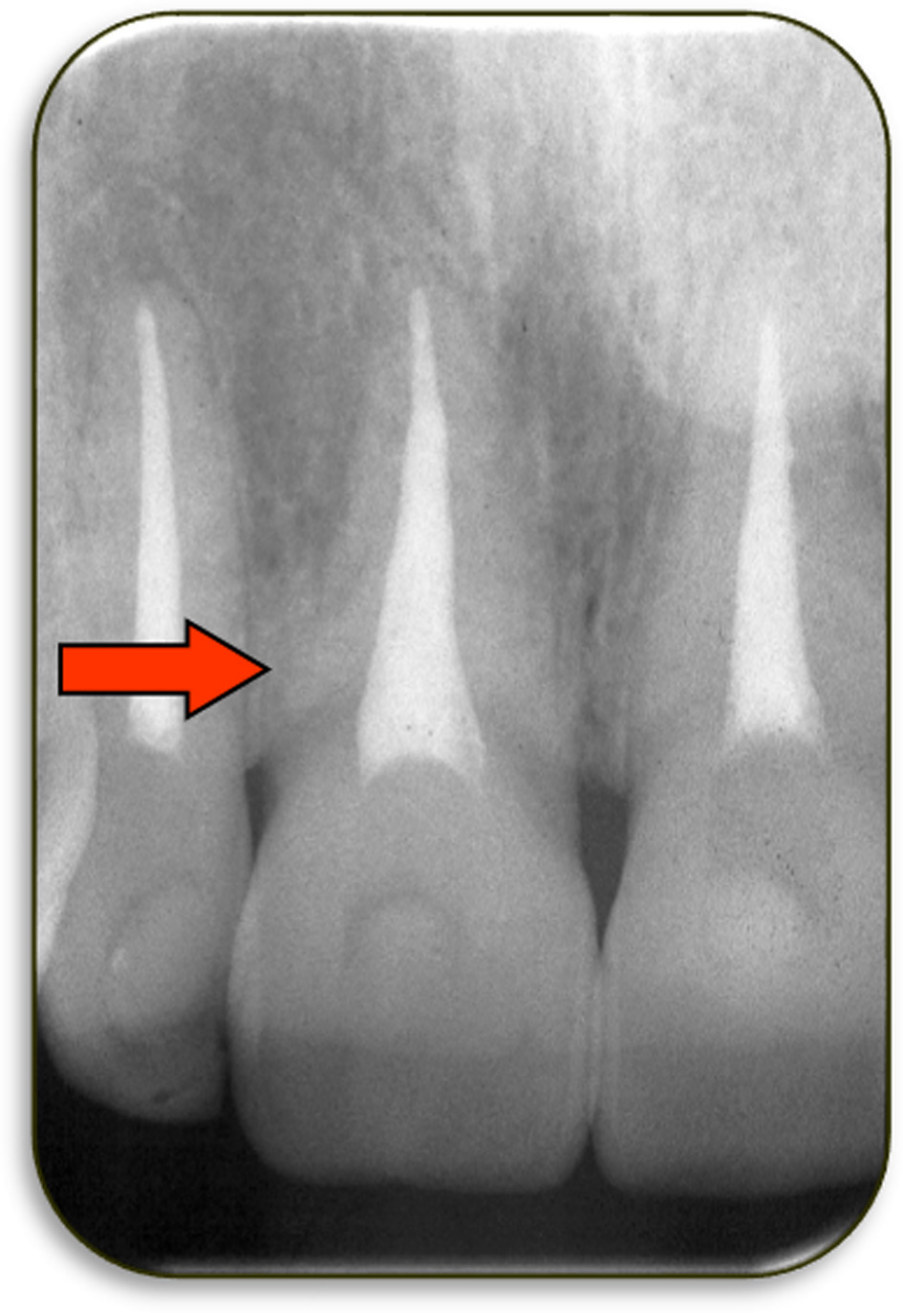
Periapical radiograph of tooth 11 which had been avulsed and replanted within a few minutes. This radiograph was taken 3 years after the injury and it shows an area of ankylosis (i.e. no periodontal ligament) but there is no external replacement resorption at this stage. The arrow indicates the region of ankylosis on the distal aspect of the cervical third of the root. This tooth also had reduced mobility and the typical percussion sound of ankylosis
